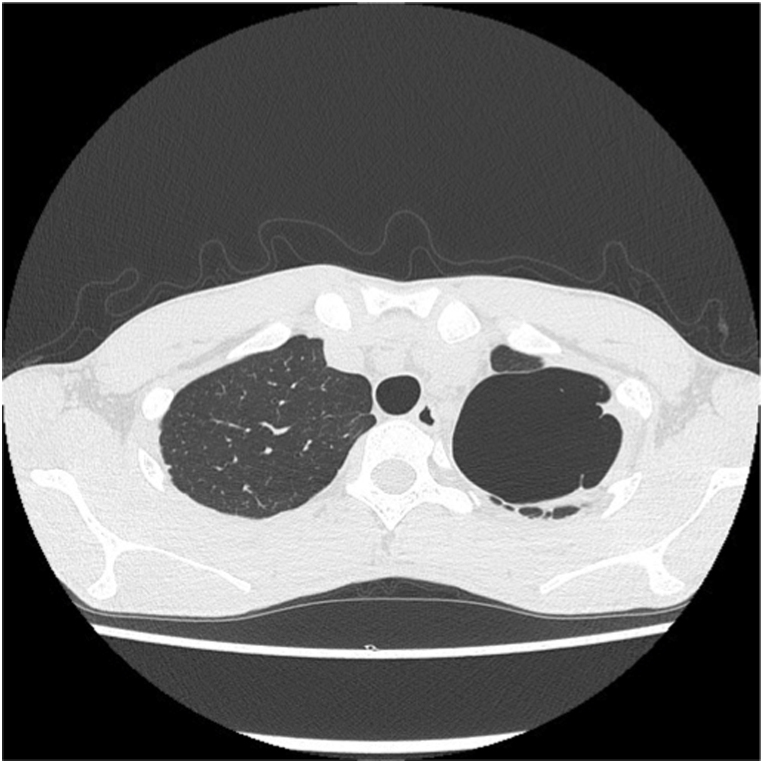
One month after the decortication, re-infection with Aspergillus occurred and persistent air leak developed. Chest computed tomography revealed parietal pleural thickening in the apical thoracic cavity.
